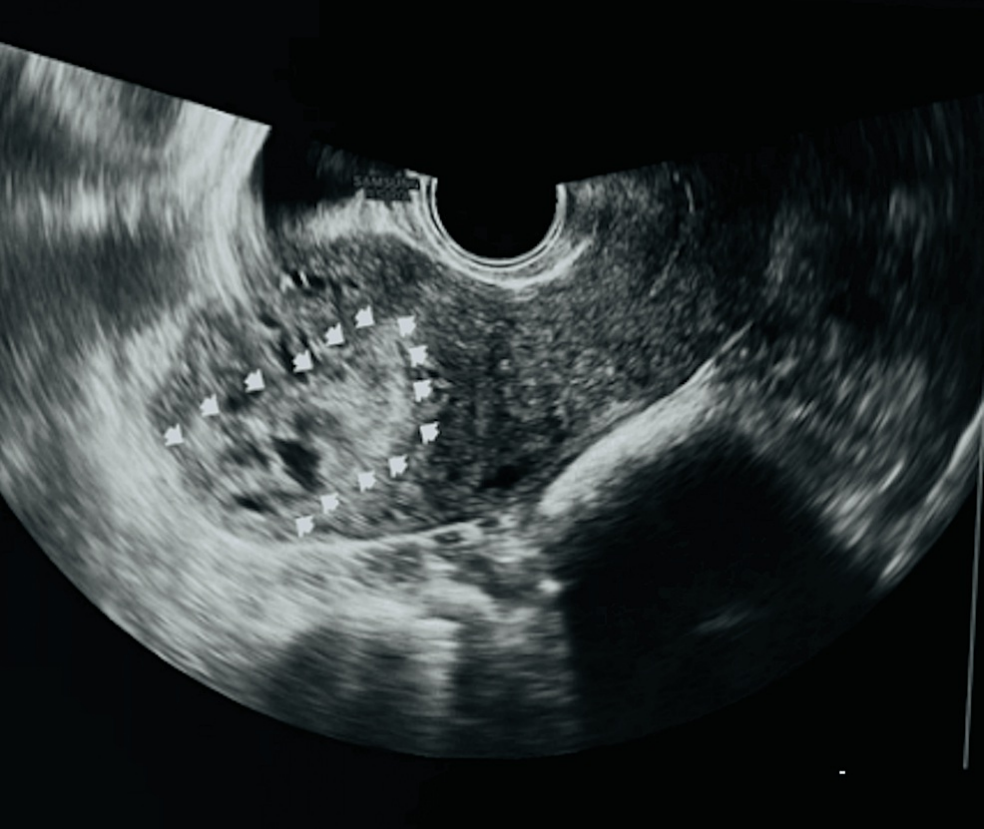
Ultrasonography showing the intra-myometrial mass
